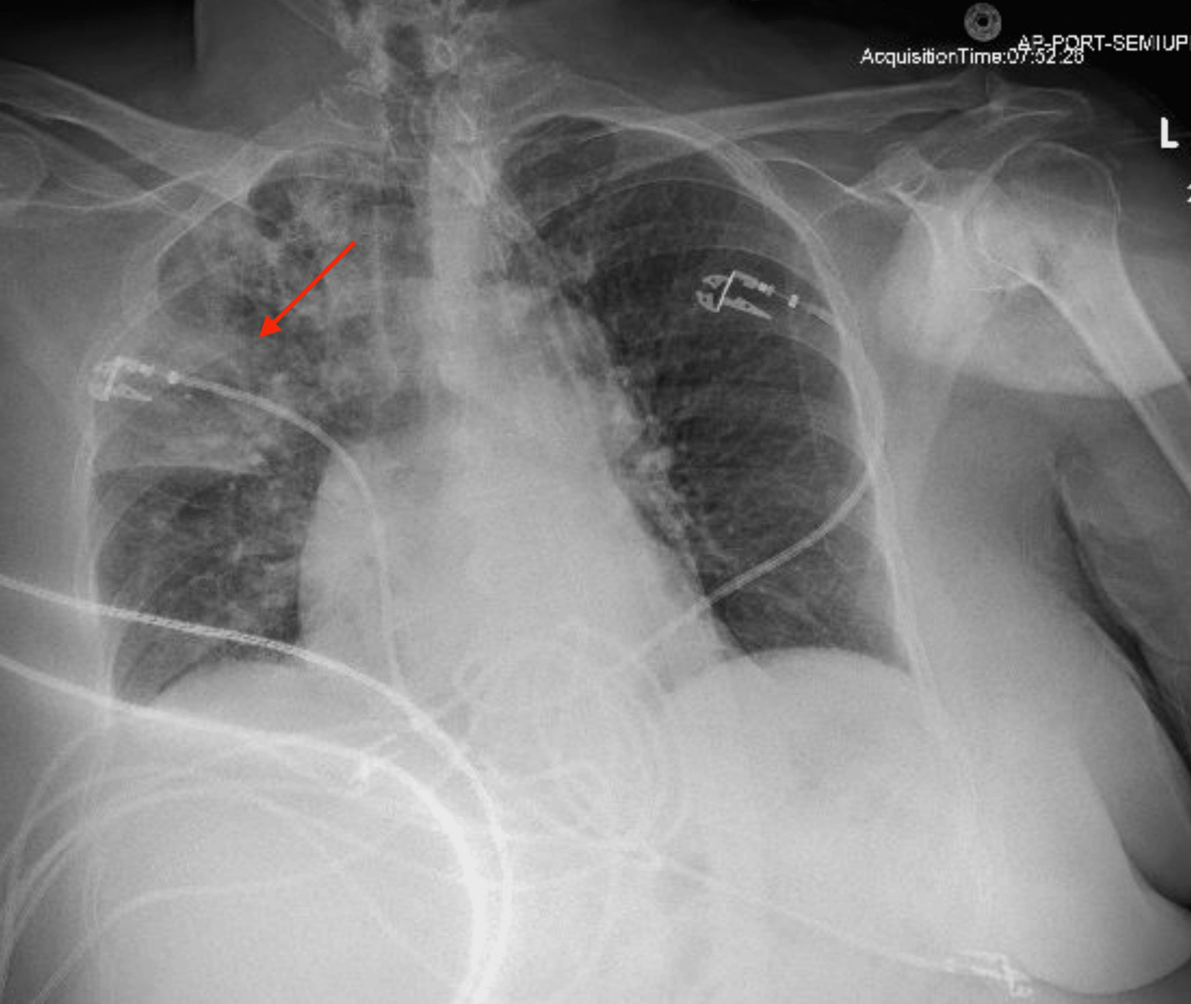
Post-thrombectomy Chest X-ray A large right upper lobe consolidation is demonstrated.
